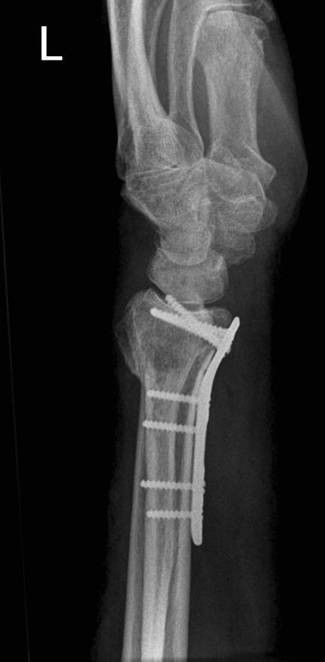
Operative treatment. Lateral wrist radiograph shows restoration of palmar inclination
